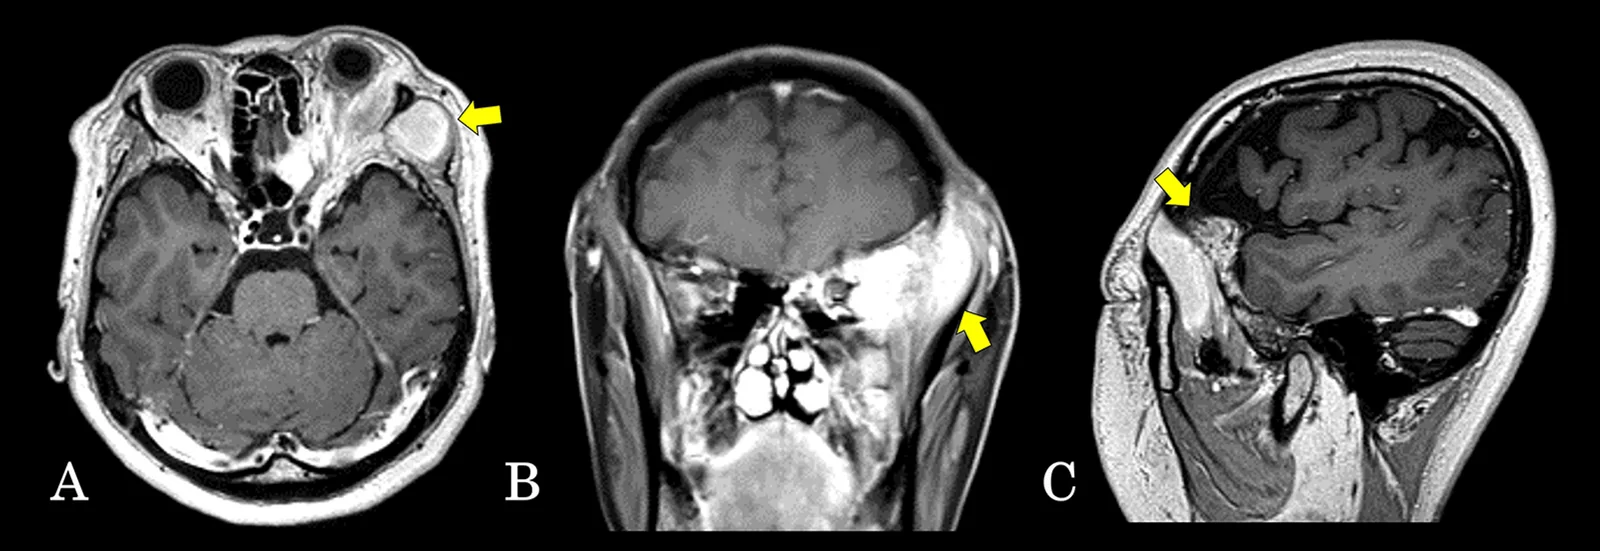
Contrast-enhanced magnetic resonance imaging findings. Arrows indicate the tumor. (A) Axial contrast-enhanced T1-weighted image. (B) Coronal contrast-enhanced T1-weighted image. (C) Sagittal contrast-enhanced T1-weighted image. These images demonstrate a well-defined, homogeneously enhancing mass centered on the left sphenoid bone, extending into the sphenoid sinus, lateral orbit, and temporal region, without evidence of brain parenchymal invasion.
